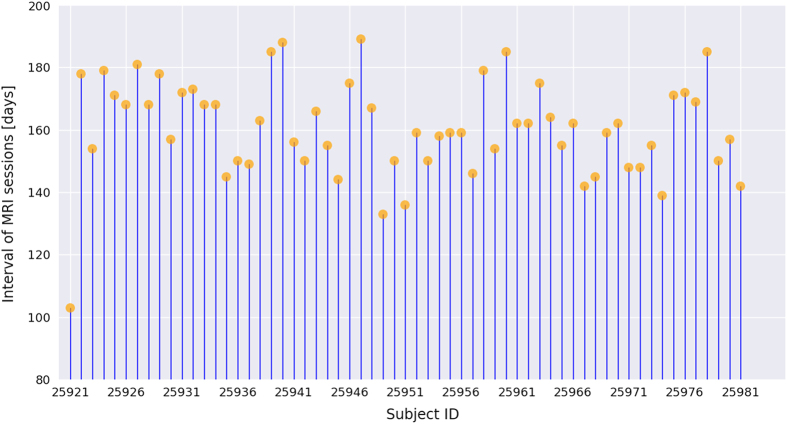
Intervals between two MRI sessions for all participants. Each vertical line corresponds to a participant, and orange disks indicate the interval length.
